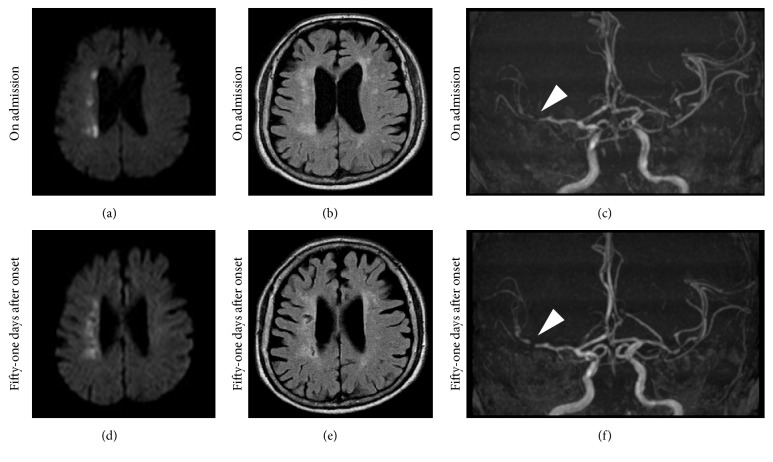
Magnetic resonance imaging (MRI) and angiography (MRA). (a) Diffusion-weighted image (DWI) on admission showed a high intensity lesion in the watershed area of the right anterior and middle cerebral arteries. (b) Fluid attenuated inversion recovery (FLAIR) on admission showed a high intensity lesion in the same area as the DWI. In addition, there were some other high intensity lesions in the deep white matter that reflected old lacunar infarctions. (c) MRA showed the stenosis of the distal portion of the right middle cerebral artery (arrowhead). (d) On the 51st day after onset, a DWI showed that the lesion was still high. (e) FLAIR on the 51st day after onset showed some of the new lesions became very low. (f) MRA on the 51st day after onset showed stenosis of the same portion of the right middle cerebral artery (arrowhead). ((a), (b), and (c)) On admission. ((d), (e), and (f)) 51 days after onset.
